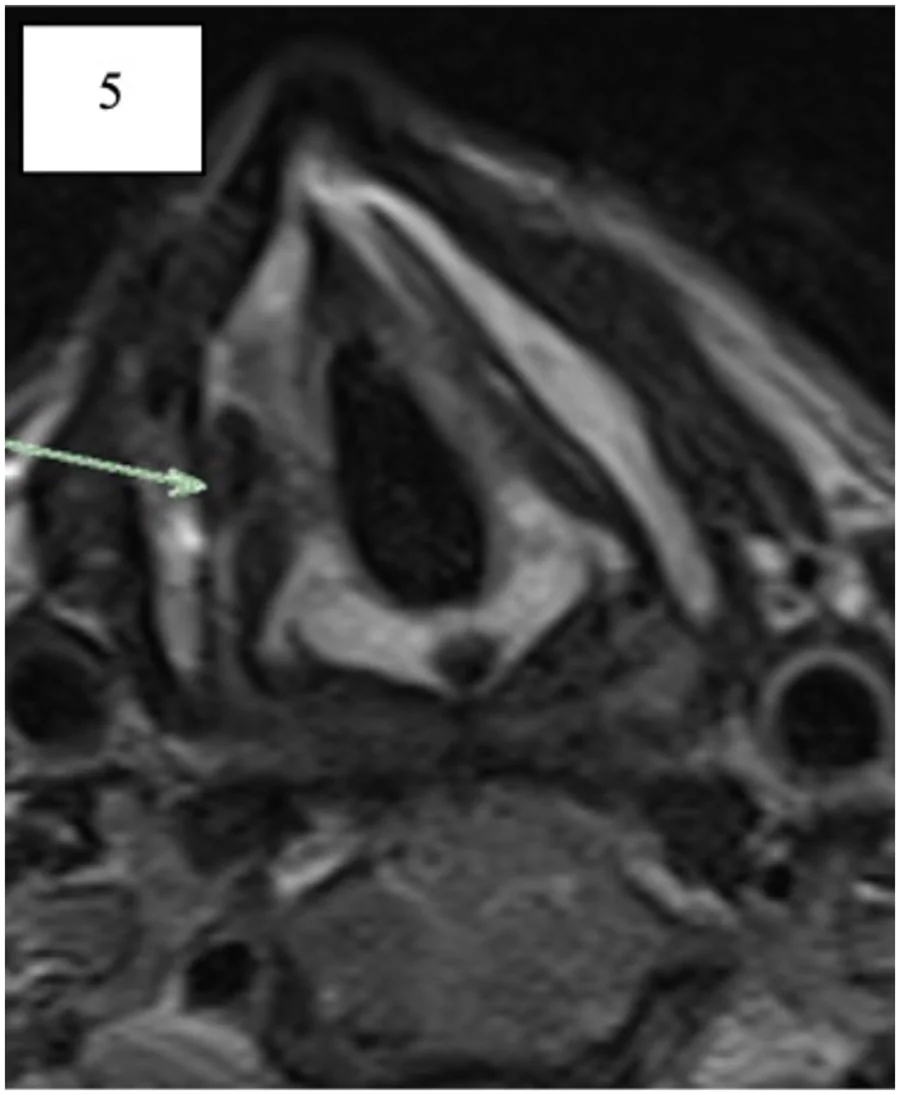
Follow-up axial T2-weighted MRI showing postoperative site. Follow-up imaging demonstrates low-signal intensity changes at the previous surgical site, consistent with postoperative fibrosis, with no radiological features suggestive of recurrent tumour.
